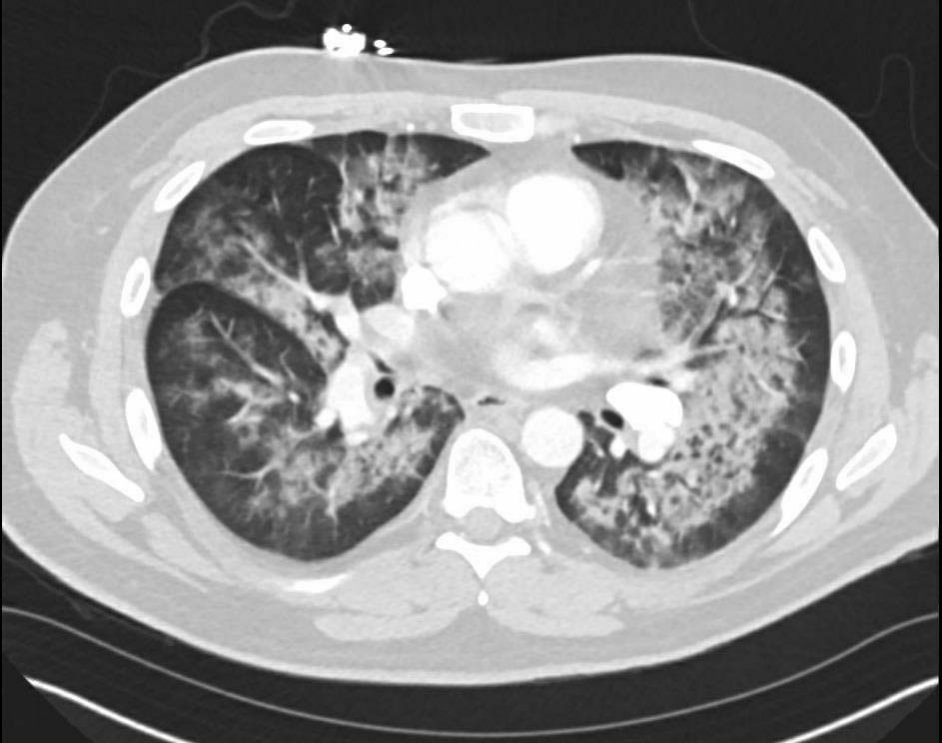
Case 1: Computed tomography angiogram of the chest shows segmental bilateral pulmonary emboli, extensive ground glass opacities, and consolidation with peripheral sparing.
